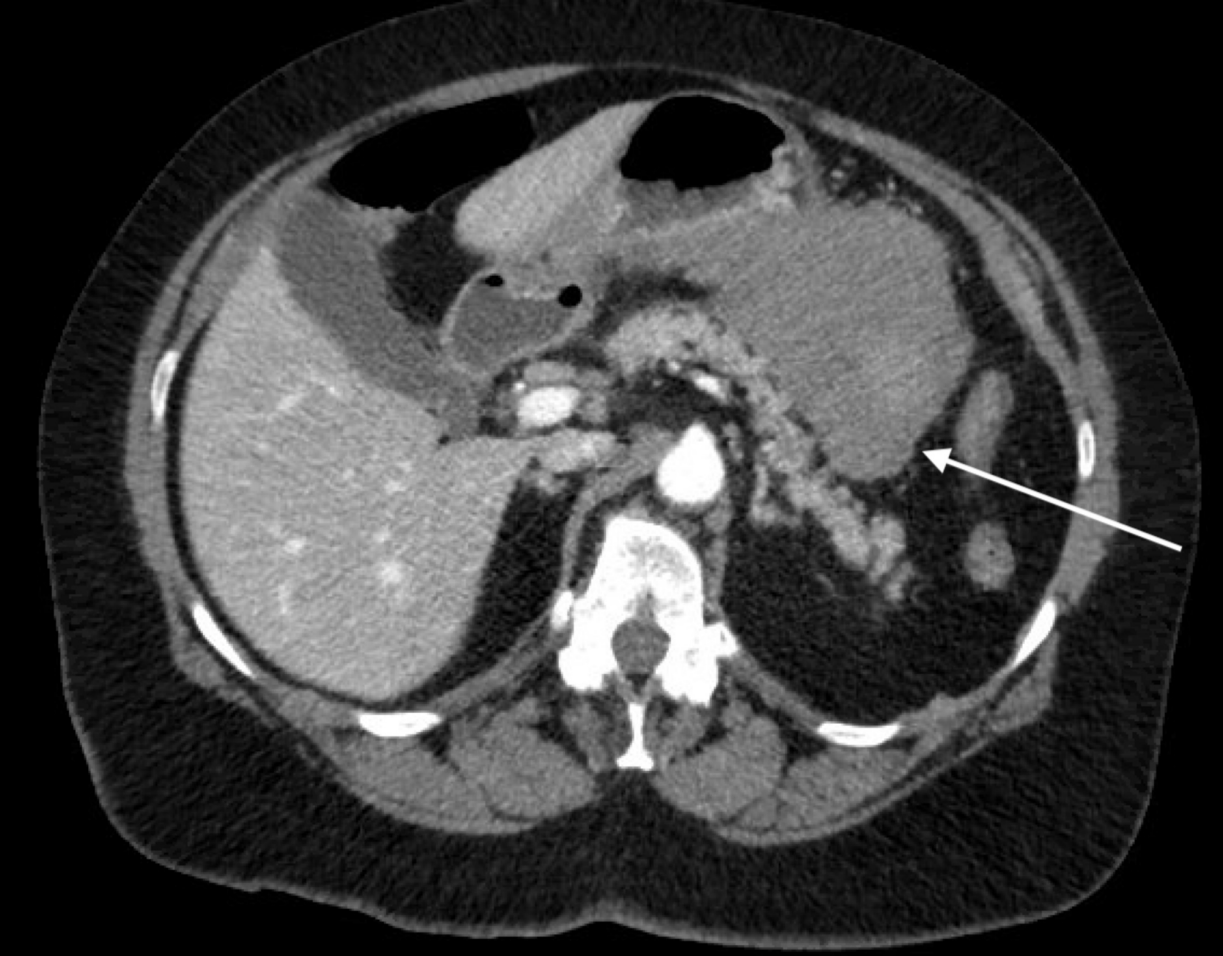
Axial CT scan showing a large amount of blood (arrow) in the lesser sac
